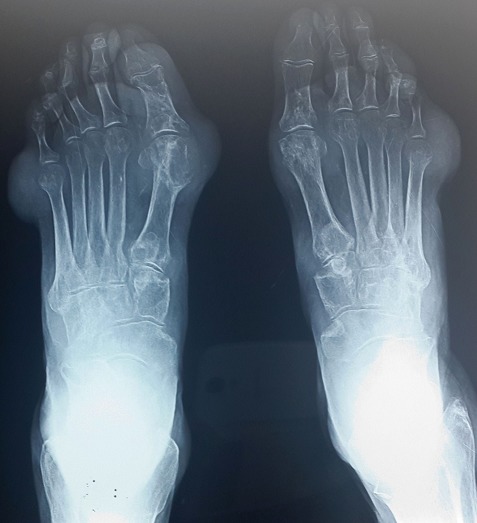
Radiography of the foot showing soft tissue swelling and destruction of the right first metatarsophalangeal joint
